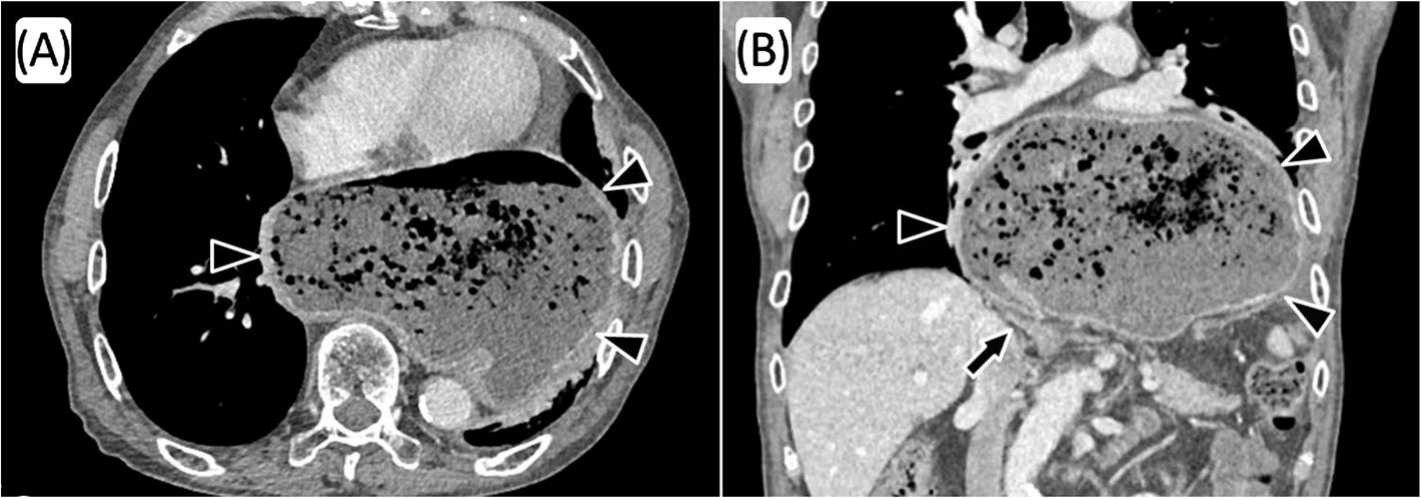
Contrast-enhanced computed tomography. A large hiatal hernia with the entire stomach (arrow heads) incarcerated through the hiatal orifice into the mediastinum, and the stomach was expanded with food remaining; in axial view (a) and in coronal view (b)
